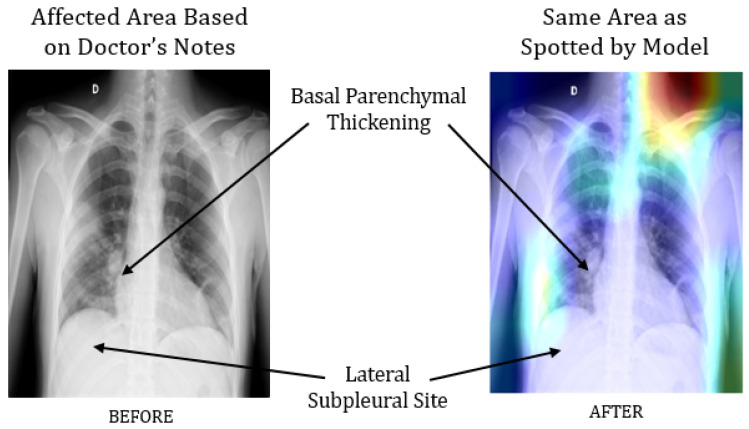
Comparison between a chest X-ray image analyzed by a doctor and a modified VGG16 model, wherein its layer “Block_4” drew particular attention to the collarbone and upper shoulder [14].
